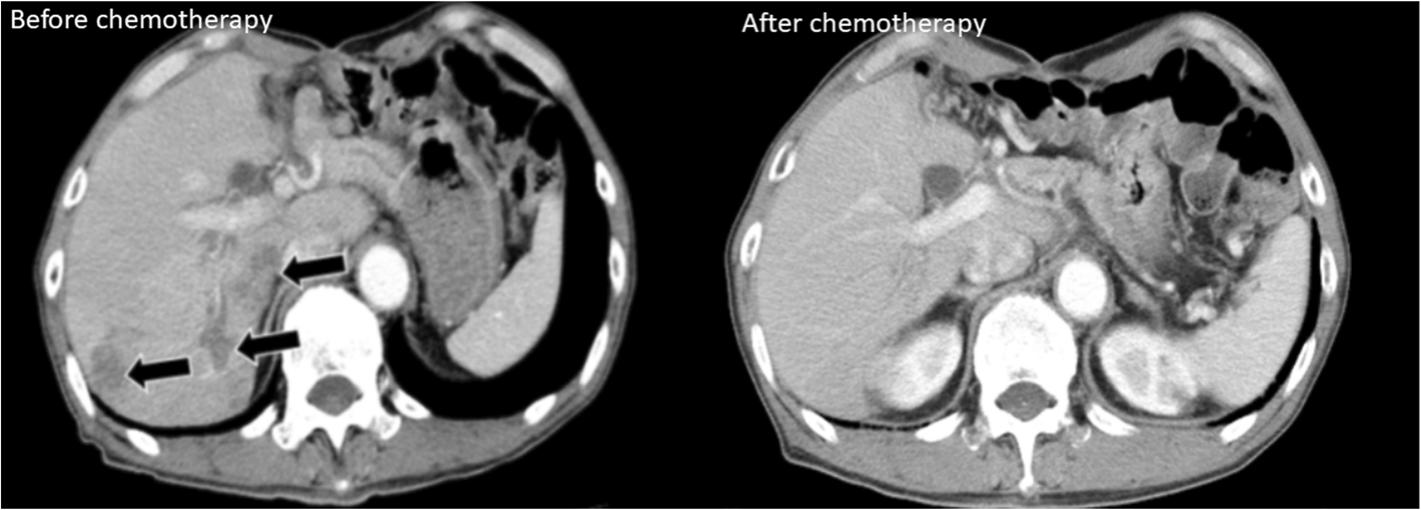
CT scan images before and after chemotherapy. Abdominal contrast-enhanced computed tomography revealed multiple low-density areas (arrows) 2 months after the second surgery. After 2 courses of chemotherapy, a CT scan revealed absence of all liver metastatic lesions
